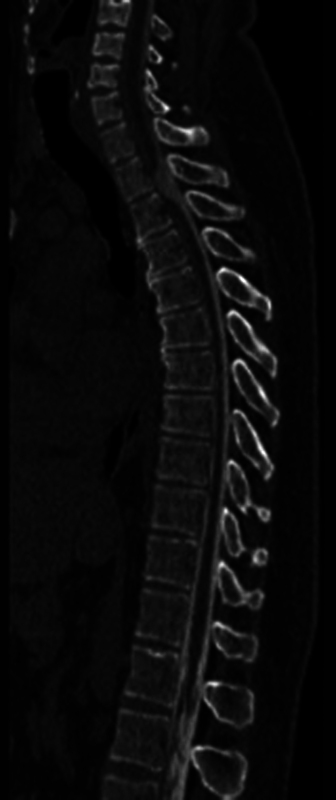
Computed tomography of the spine – sagittal bone window.
